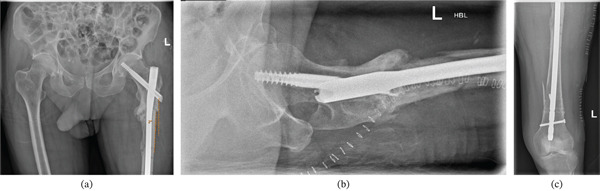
(a) Early postoperative anteroposterior radiograph showing cephalomedullary nail insertion and the satisfactory valgus alignment (measured 2°) in comparison to preoperative (Figure 11) radiographic imaging (measured angle 13°), (b) early postoperative lateral radiograph of left femur following revision fixation with cephalomedullary nail insertion and valgus correction. (c) early postoperative anteroposterior radiograph of left femur following revision fixation with cephalomedullary nail insertion, valgus correction and distal locking screw.
